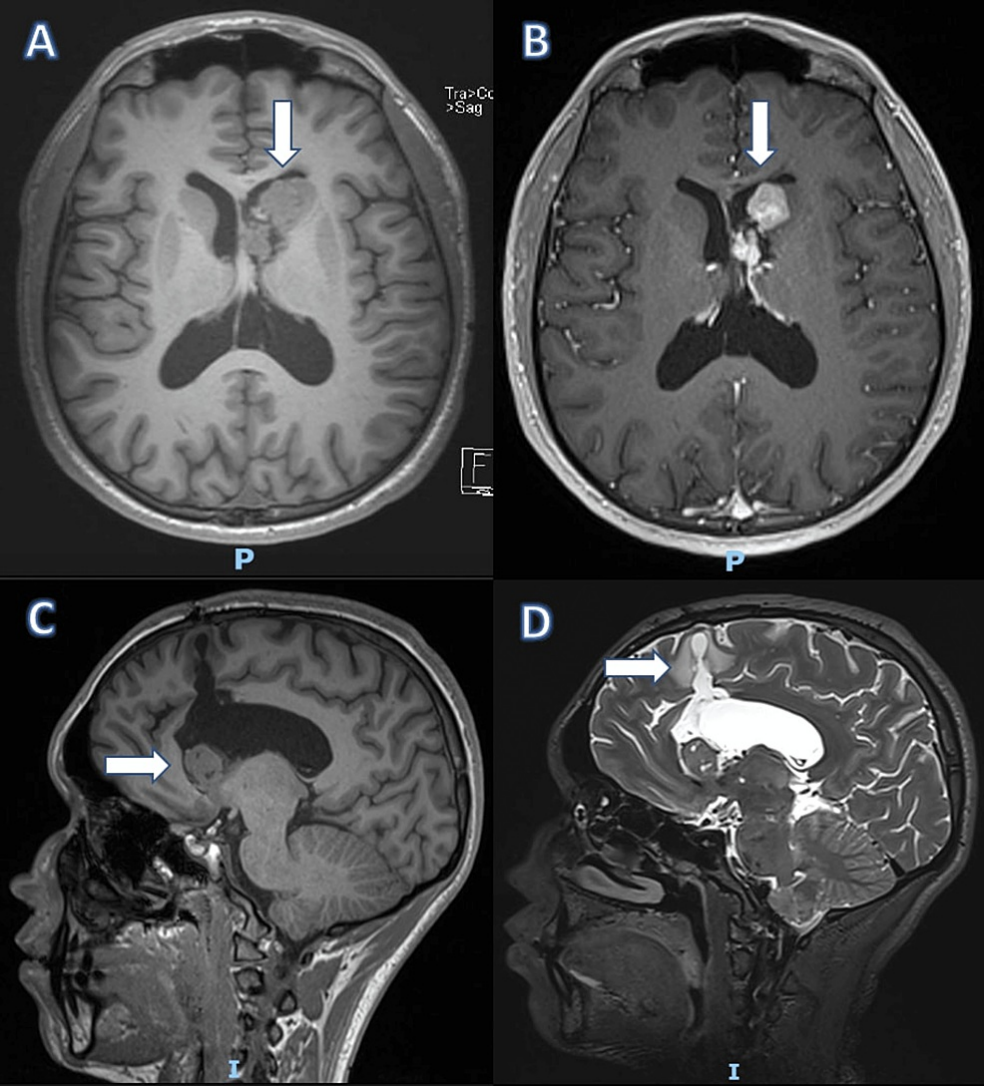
Cranial magnetic resonance imaging in 2019 and 2021 (A) Axial, T1, 2019. (B) Axial, FSPGR (fast spoiled gradient-echo) + gadolinium, 2021. (C) Sagittal, FSPGR, 2019. (D) Sagittal, T2, 2021. (A,C) An intraventricular, isointense, multilobulated tumor (white arrow) at the anterior horn of the left lateral ventricle was observed. (B) With gadolinium, a 3.1 × 1.7 × 2.0 cm tumor exhibited intense and heterogeneous enhancement (white arrow). It was attached to the septum pellucidum and slightly displaced and obliterated the left foramen of Monro. (D) Post-surgical changes were identified (white arrow). When comparing the 2019 and 2021 studies, no substantial changes were observed.
